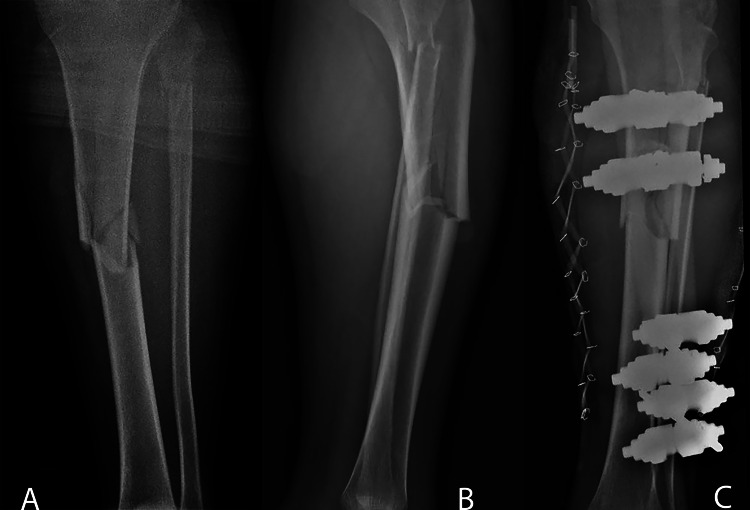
A: Anteroposterior radiograph demonstrating comminuted tibial shaft fracture and proximal fibula fracture. B: Lateral radiograph demonstrating comminuted tibial shaft fracture and proximal fibula fracture. C: Post-operative anteroposterior radiograph showing the placement of an external fixator to the tibial shaft fracture as well as the presence of rubber band lace-up technique on both sides of the leg.
